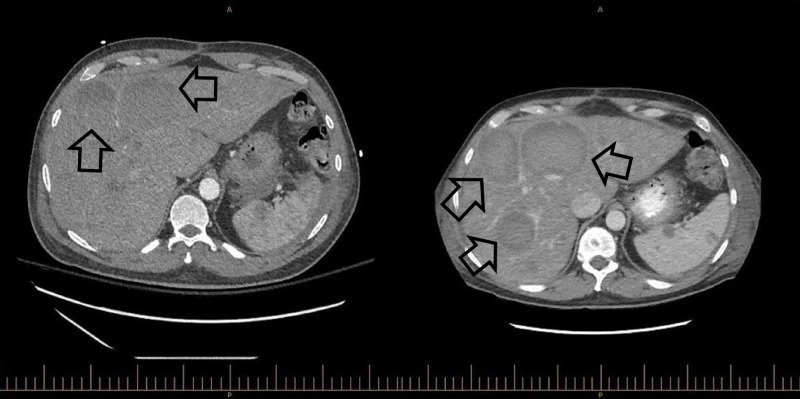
Side-by-side CT abdomen and pelvis with and without contrast imaging The study on the left was conducted at initial presentation and shows two liver lesions. The image on the right was performed one month later and demonstrated rapid disease progression with extensive metastatic disease infiltrating the parenchyma of the liver. CT: computed tomography
